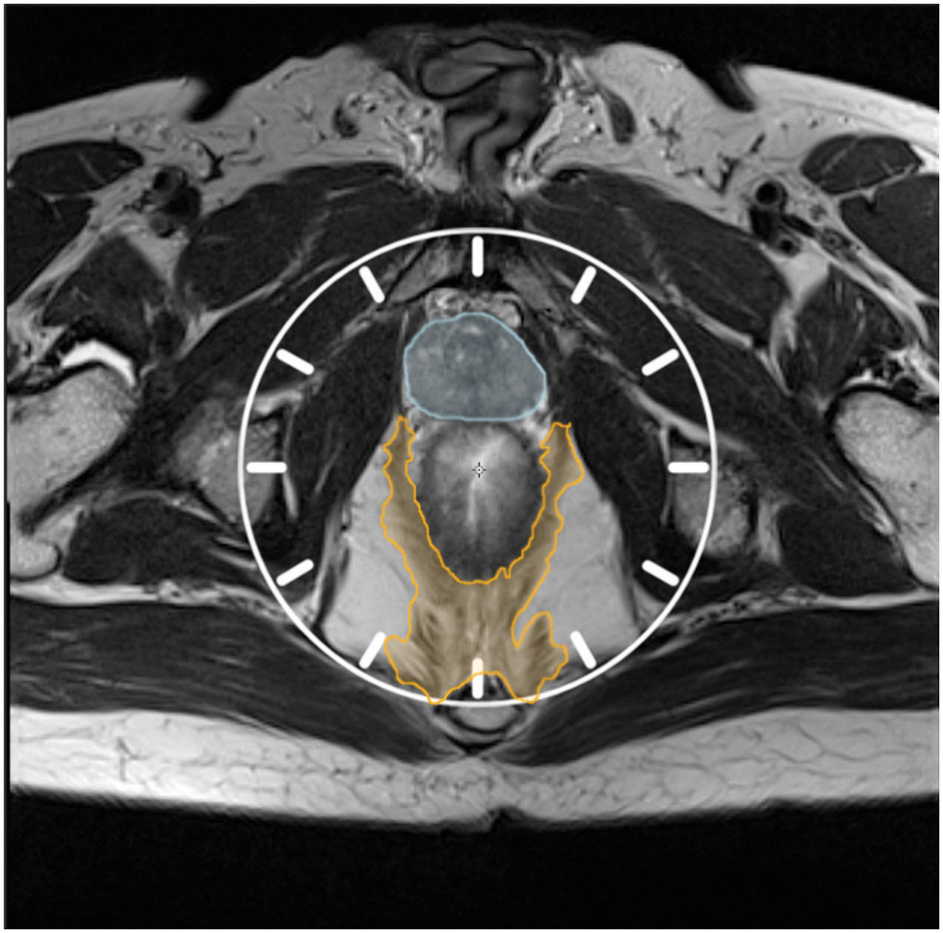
Visualizing the axial plane of T2w MRI as the face of a clock. Orange hatching: Pelvic diaphragm. Blue hatching: Prostate.
